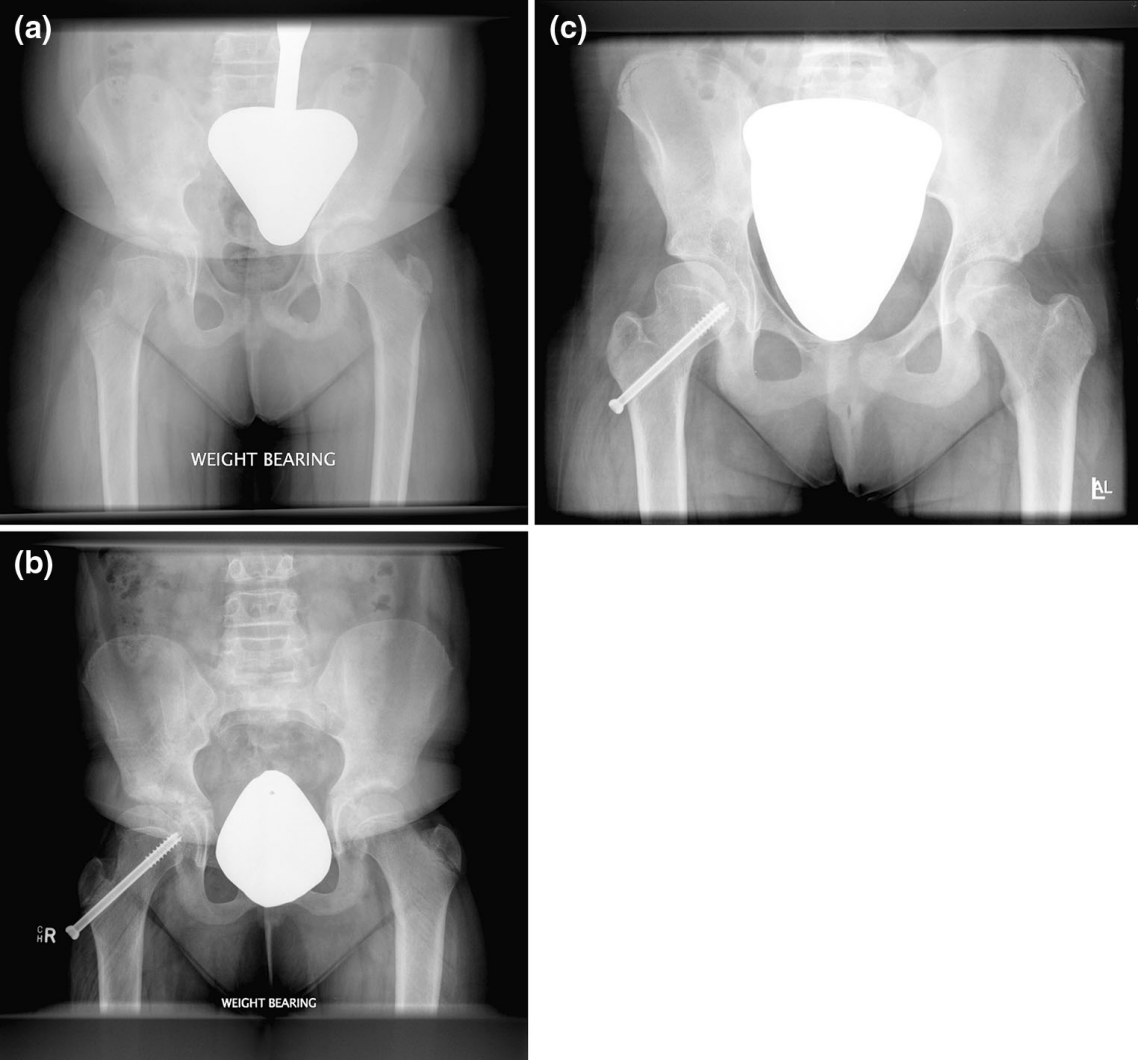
a Preoperative radiograph of an 8-year-old patient with right DDH. b Pelvic radiograph following insertion of a right transphyseal screw. c Pelvic radiograph 5 years after the insertion of the transphyseal screw
